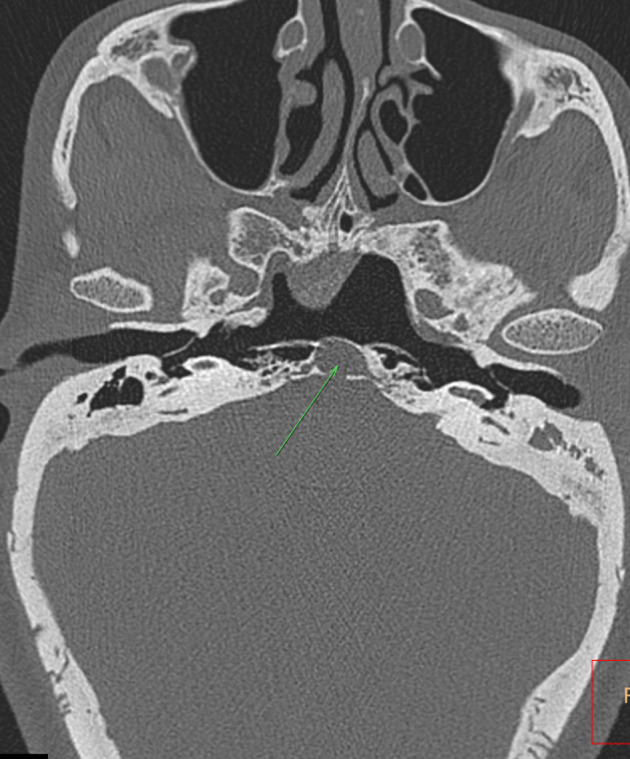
Transverse high resolution CT scan through the base of the temporal bone demonstrating large pharyngotympanic tubes with clear communication with the middle ear. Note absent carotid canals and a round defect in the body of the Clivus (arrow in Figure 1).
